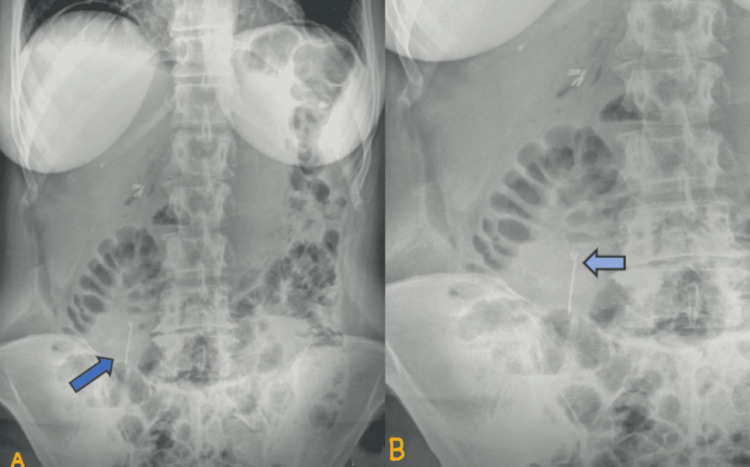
(A) Abdominal X-ray showing the foreign object (blue arrow) that was detected on the CT scan and exhibiting the futures of a sewing needle. (B) Closer view of the abdominal X-ray pointing out the sheath of the needle (light blue arrow)
